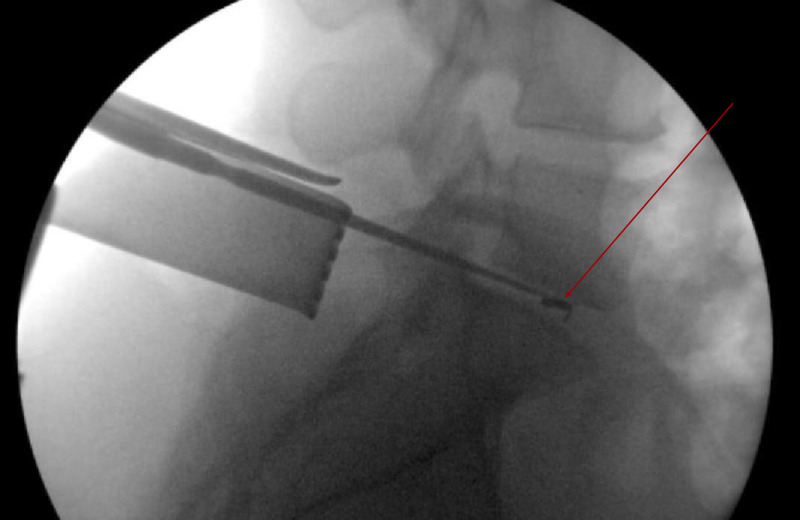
Lateral Radiograph of Blunt Nerve Hook Palpation of Broken Rongeur
